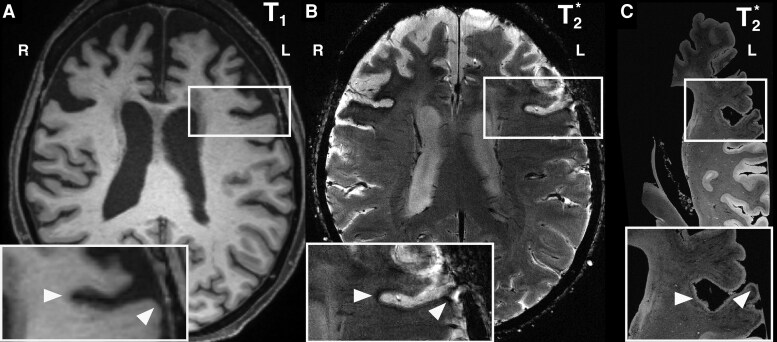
Correspondence of antemortem 7T T_2_*w MRI hypointensity with iron-rich gliosis on post-mortem ex vivo 7T T_2_*w MRI. (A) Patient #4 with naPPA antemortem three Tesla T1-weighted (3T_1_w) MRI, (B) antemortem 7T T_2_*w MRI and (C) post-mortem *ex vivo* 7T2*w MRI axial views and zoomed regions (insets). Note the correspondence of hypointense cortical signal in BA8 on *in vivo* 7T T_2_*wMRI (B arrows) to iron-rich hypointense banding on corresponding *ex vivo* 7T2*w MRI (C arrows), while 3T_1_w MRI does not show hypointensity and instead shows reduced GM/WM contrast (A arrows).
